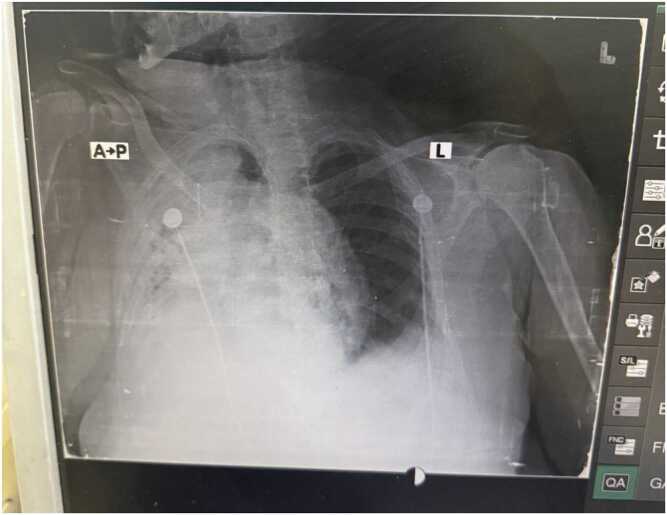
Chest X-ray during hospitalization (approx. 2nd September): persistent diffuse opacities, worsening consolidation.
